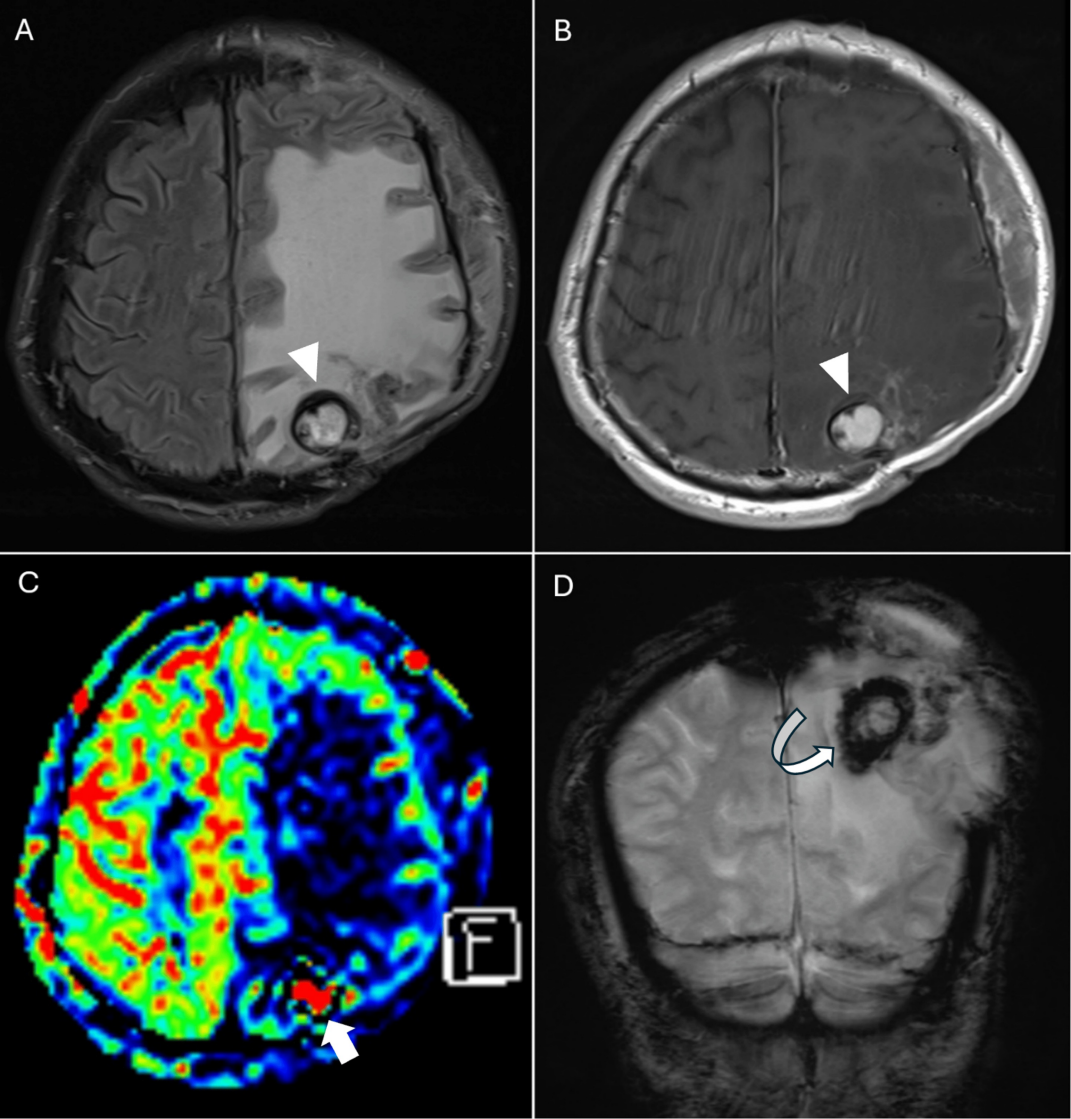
Cranial MRI obtained 15 years after stereotactic radiosurgery and two weeks after decompressive craniectomy and intravenous steroid treatment MRI: magnetic resonance imaging (A) Axial T2-weighted image and (B) axial T1-weighted image with contrast show enlargement of the left parietal enhancing lesion with a peripheral low-signal rim (arrowheads) and persistent progressive vasogenic edema. (C) Axial arterial spin labeling (ASL) perfusion imaging demonstrates focal hyperperfusion (arrow) within the lesion and surrounding hypoperfusion corresponding to the edematous areas. (D) Coronal T2*-weighted gradient-echo (GRE) image reveals a low-signal (dark) rim (curve arrow) around the lesion, consistent with hemosiderin deposition or prior hemorrhage
